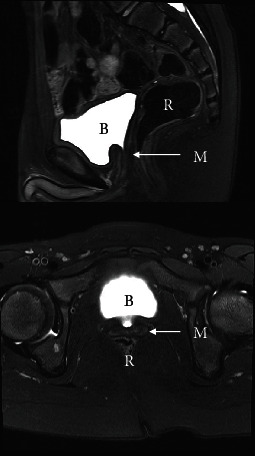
Magnetic resonance imaging (MRI) sagittal and axial views showing Müllerian remnant. Key: M: Müllerian remnant; B: bladder; R: rectum.
